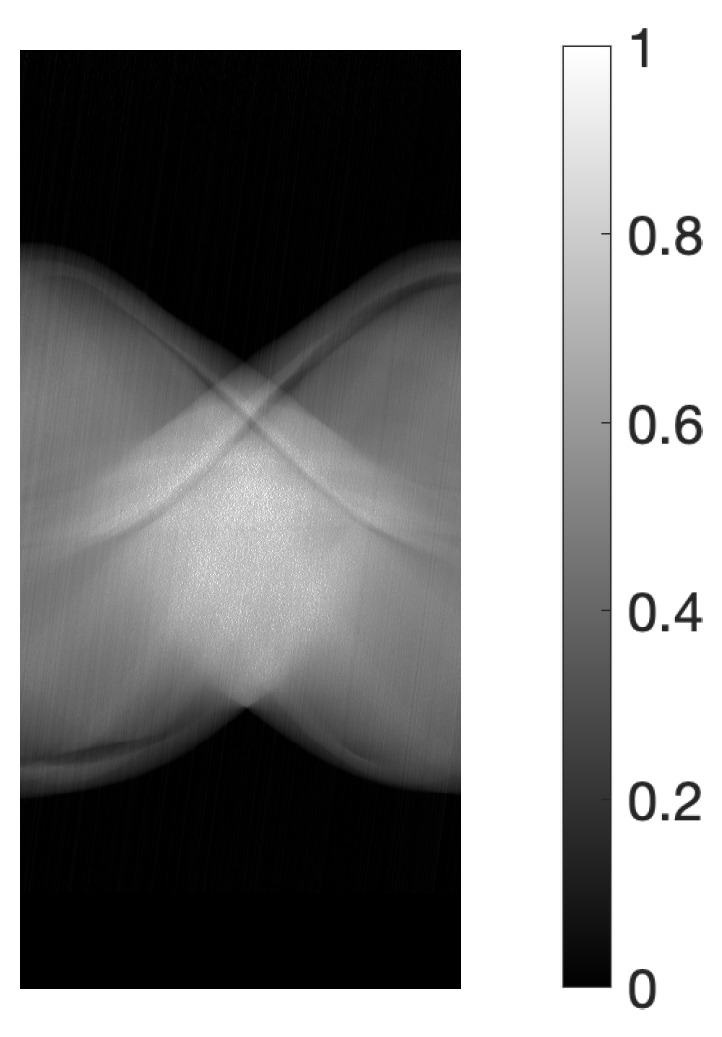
Sinogram obtained from X-ray CT scanner.
